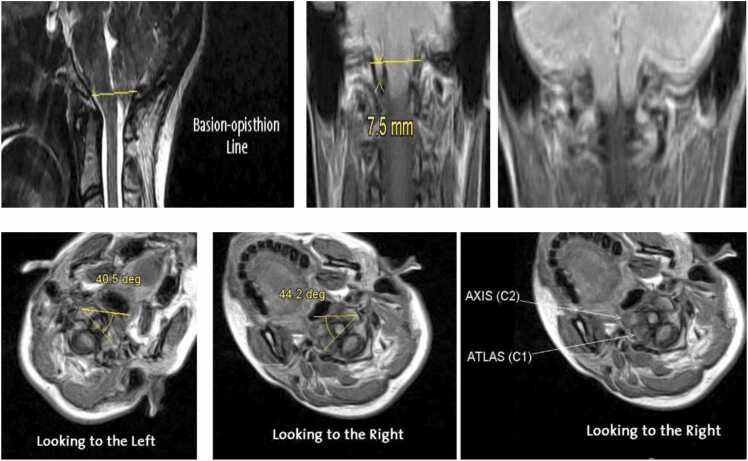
Upright MRI at the cranio-cervical junction showing cerebellar tonsillar ectopia, with the right tonsil descending ∼7 mm below the foramen magnum.
